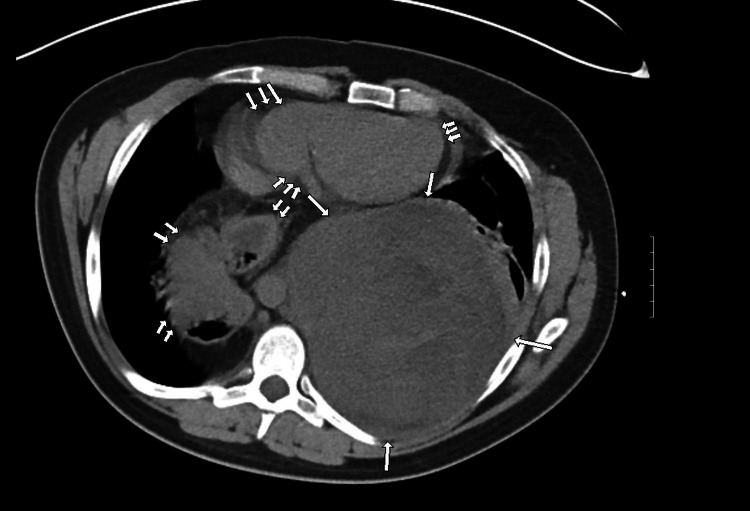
Non-enhanced chest CT axial image of the lower chest with a soft tissue window (with arrows): a large intrathoracic extrapulmonary mass compressing the heart is seen (single arrow). An incidental hiatal hernia is also visualized in the right lung space (double arrows). The heart is compressed anteriorly by the mass against the chest wall (triple arrows). The abnormality does not contain fat density when compared to abdominal fatty regions. Hounsfield units (HU) range from 5 to 30 HU with an average of 25 HU across the abnormality.
